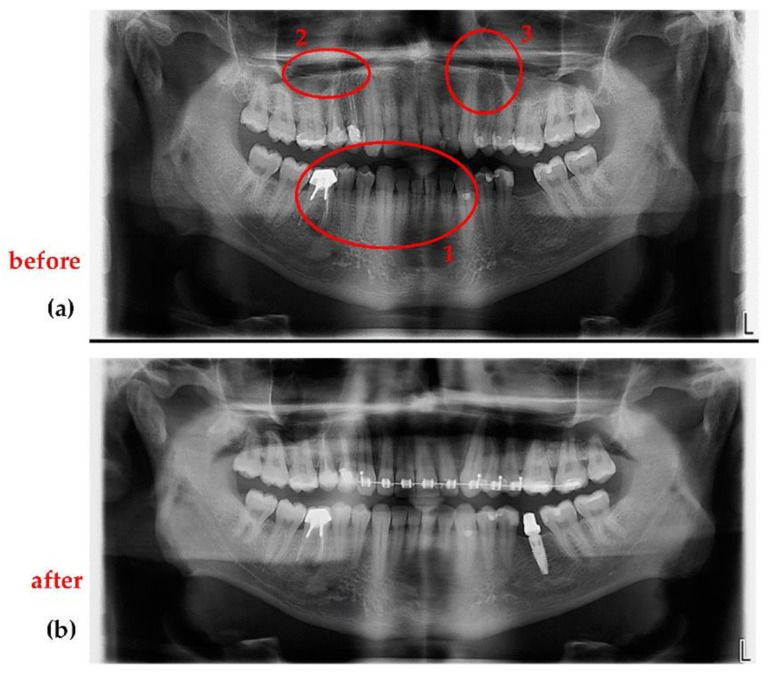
Panoramic radiographs performed on the same patient (F.C., male, 29 years old) (a) before and (b) after the optimization.
